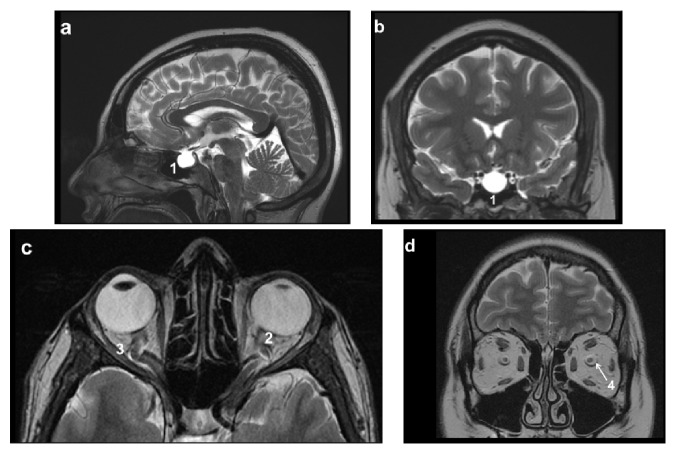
Signs of idiopathic intracranial hypertension on cerebral MRI, T2-weighted images. 1: empty sella, 2: flattening of the posterior aspect of the globe, 3: tortuous optic nerve, and 4: distention of the perioptic subarachnoid space.
